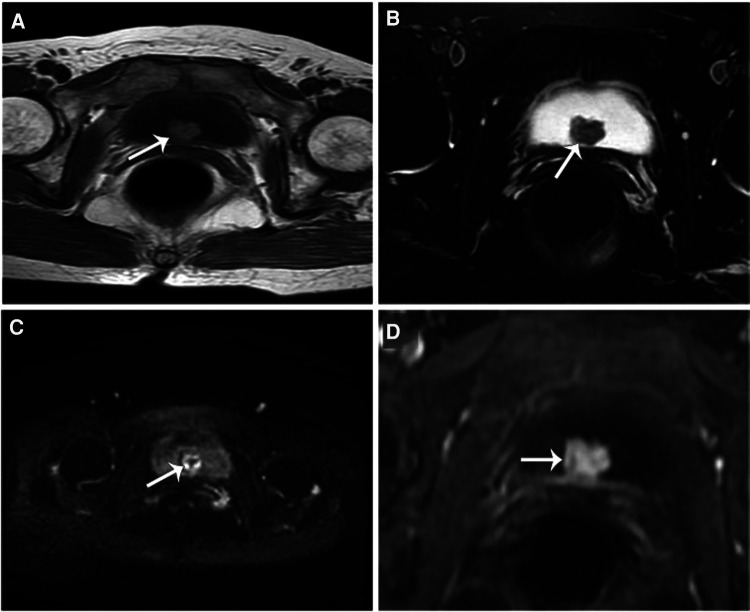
T1wt1 image (A) and STIR images (B) showed cauliflower-like soft tissue signal nodular shadow (white arrow) in the posterior bladder wall, which was isosignal compared with that of the muscle. It had a wide basal connection with the bladder wall. High signal nodular shadow on DWI (C), with significant inhomogeneous enhancement on enhancement scan (D).
